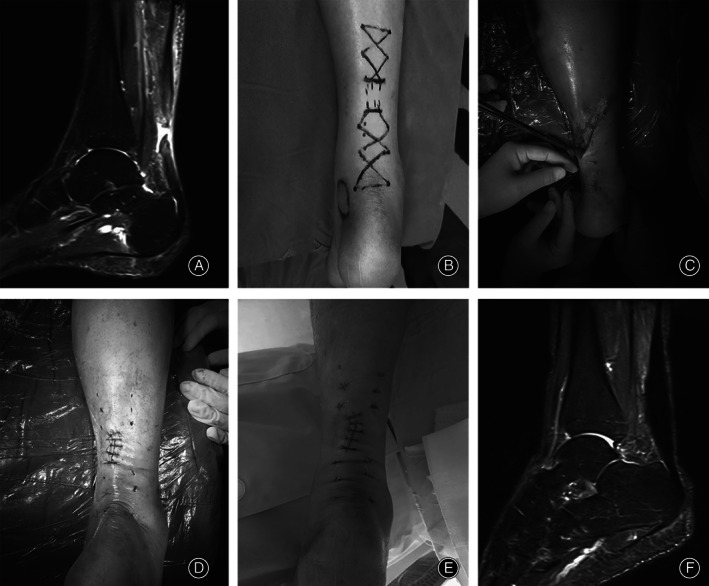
A 44‐year‐old middle‐aged man suffered a rupture of the Achilles tendon after a sprain. (A) Preoperative right lower limb MRI showed rupture of Achilles tendon; (B) draw the operation route before operation; (C) Suture Achilles tendon with the help of epidural puncture needle; (D) Close the incision and complete the operation; (E) On the second day after operation, the surgical incision was small and the patient was very satisfied; (F) Postoperative MRI showed that the Achilles tendon anastomosed well, and during the postoperative follow‐up, it was found that the patient returned to normal work and sports.
